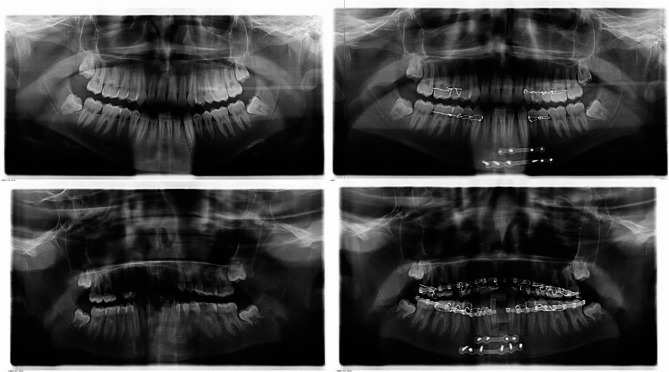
Pre& post-operative radiographic evaluation
